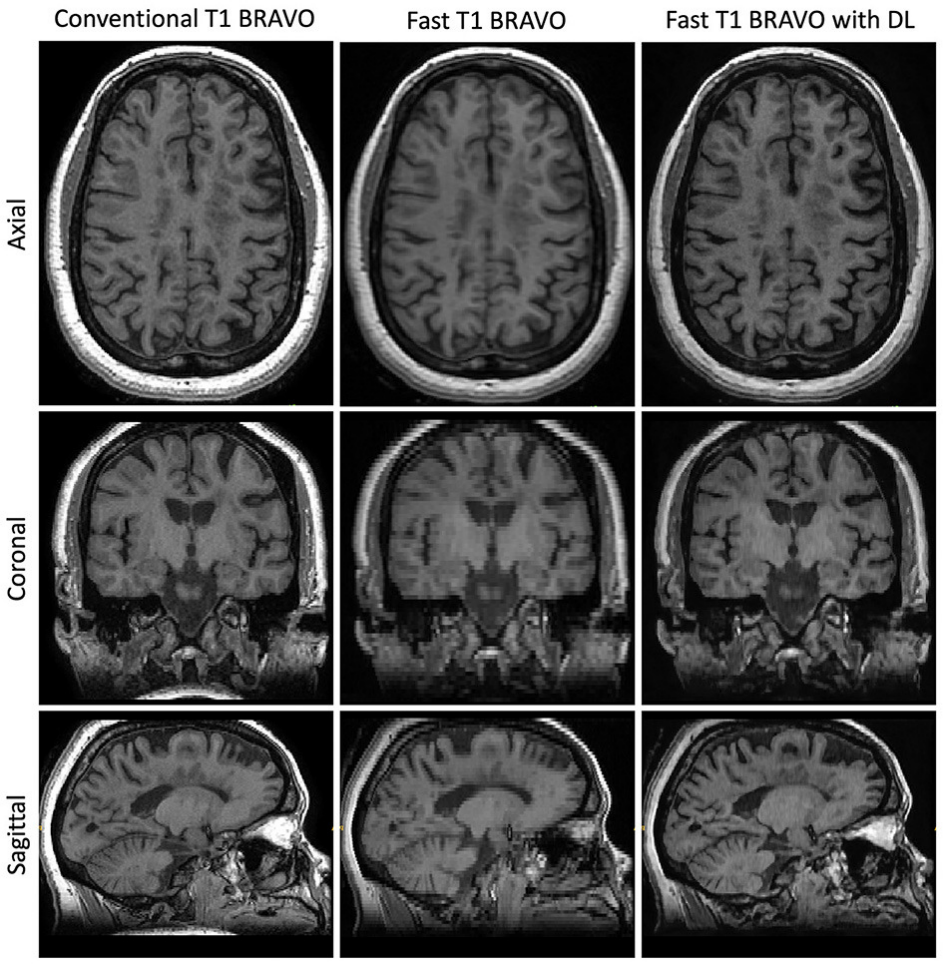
Representative T1w images. Central slices in a similar position for the acquisitions using the three methods: Conventional T1w BRAVO (2:57 min total acquisition time, 220 × 220 × 126 matrix size), fast T1w BRAVO (1:13 min total acquisition time, 220 × 128 × 64 matrix size), and fast T1w BRAVO with DL (same acquisition time and matrix size as the fast T1w BRAVO). BRAVO is the T1-weighted sequence for brain volume imaging on GE MR scanner.
